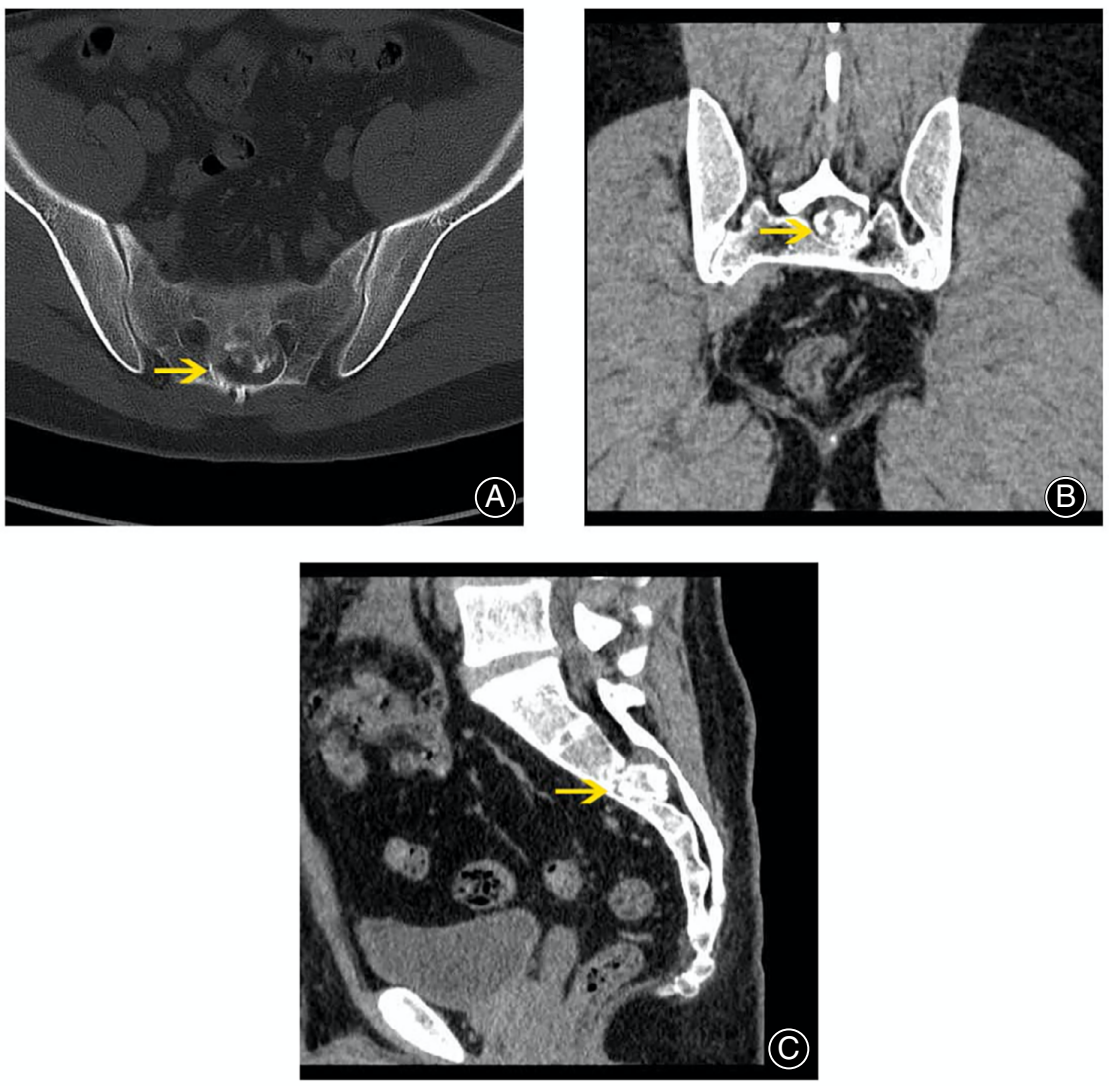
(A) The transverse CT scan showed the lesion in S2 level, an osteolytic lesion with calcified part; (B) the coronal CT scan showed the relationship between the tumor and sacral nerve; (C) the sagittal CT scan showed the lesion's location in AP direction
